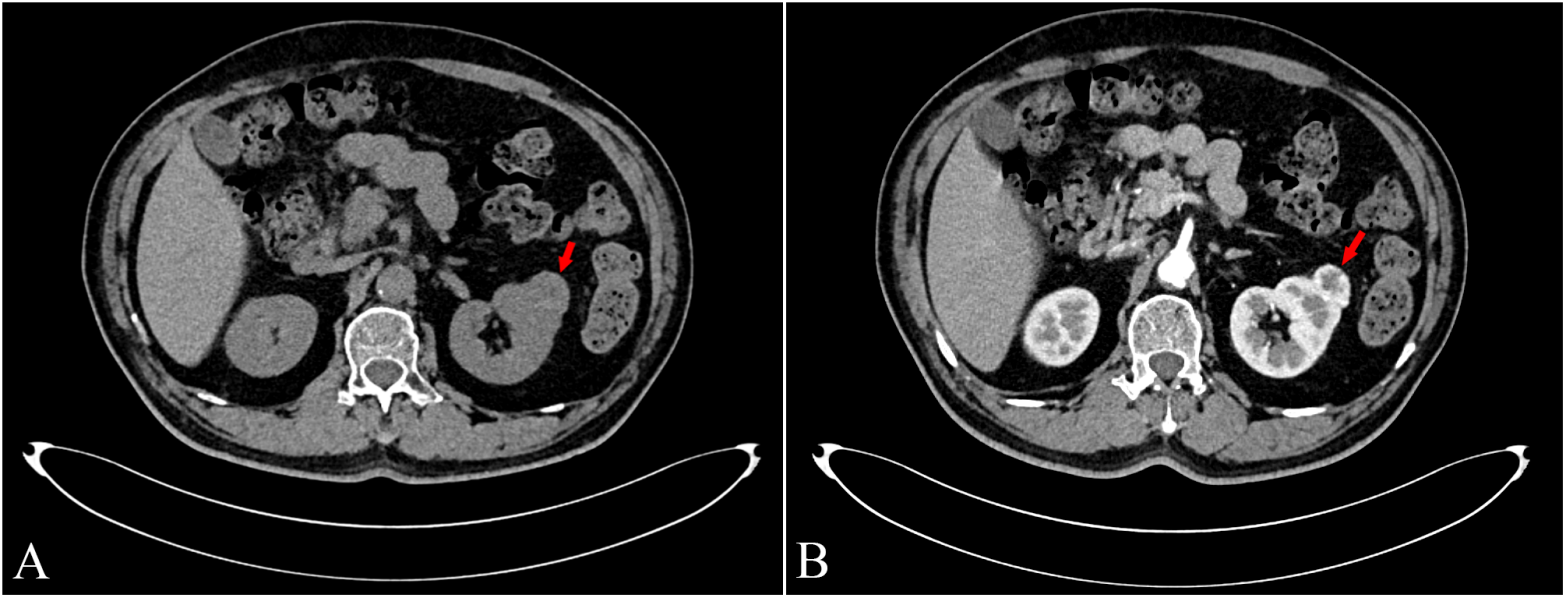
Abdominal contrast-enhanced CT scan of the kidney tumor and tumor macroscopy. An abdominal contrast-enhanced CT scan revealed a soft-tissue density protuberance with a diameter of approximately 28 mm in the left kidney (A, red arrow). In the arterial phase, the tumor exhibited slightly uneven enhancement, and the capsule was significantly enhanced (B, red arrow).
